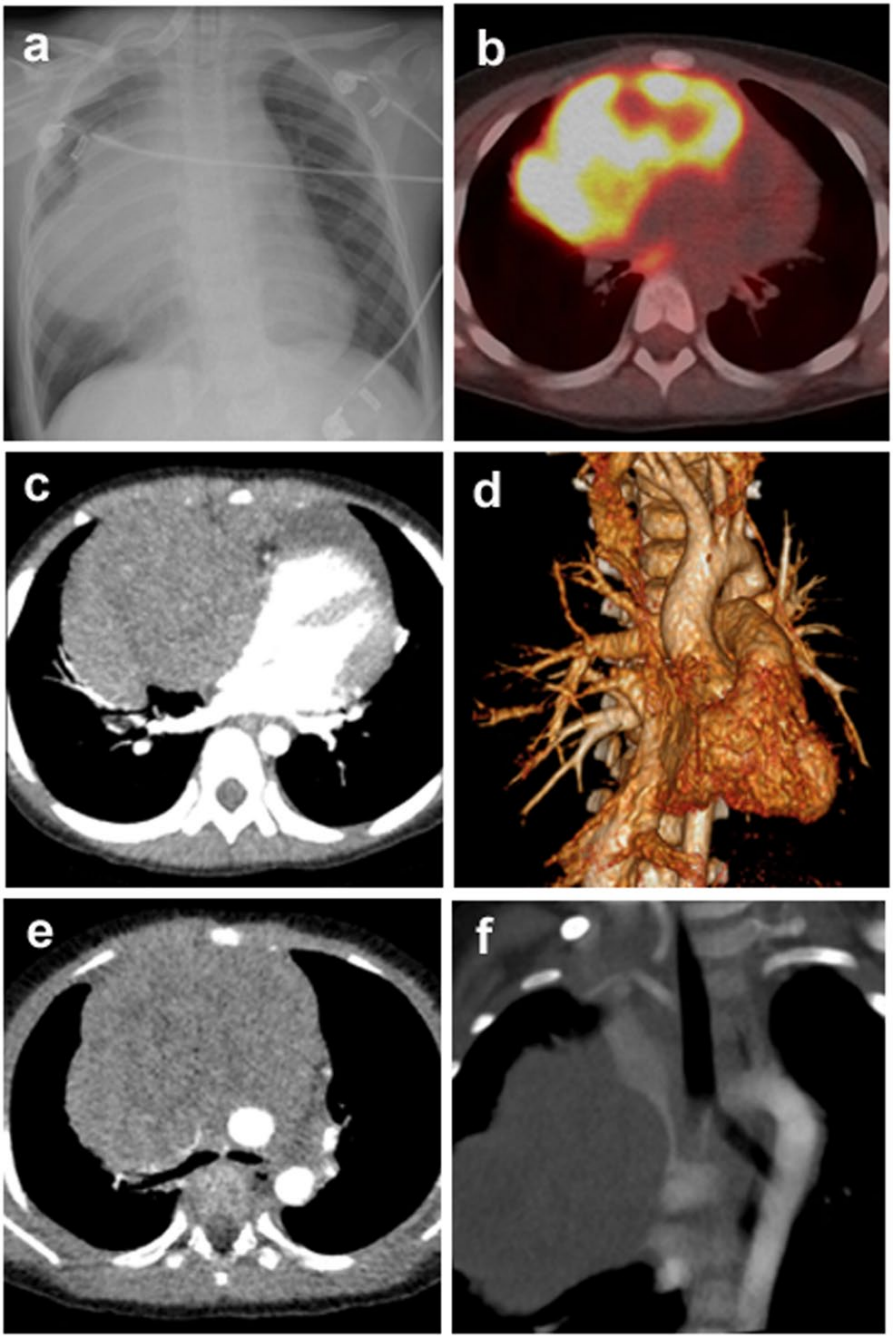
Radiological characteristics of T-LBL/T-ALL involving the mediastinum, case 1. (a) Chest X-ray image shows a large mass in the mediastinum. (b) PET-CT image shows increased FDG activity in the mediastinum. (c) Axial contrast-enhanced CT scan shows the compression of pericardiaum. (d) Three-dimensional volume rendered image of contrast-enhanced CT exhibits the compression of pericardiaum. (e) Axial contrast-enhanced CT scan shows vascular compression and tracheal encasement of the mass. (f) Maximal intensity projection of contrast-enhanced CT reveals the encasement of superior vena cava.
